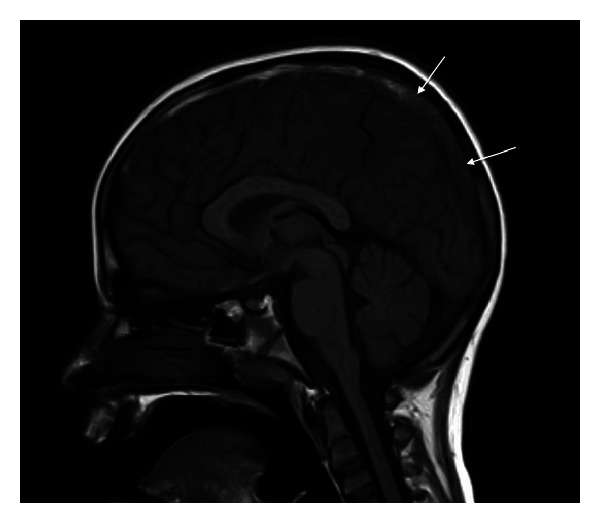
Sagittal T1-weighted MRI reveals low-attenuating thrombus (arrows) within superior sagittal sinus.
